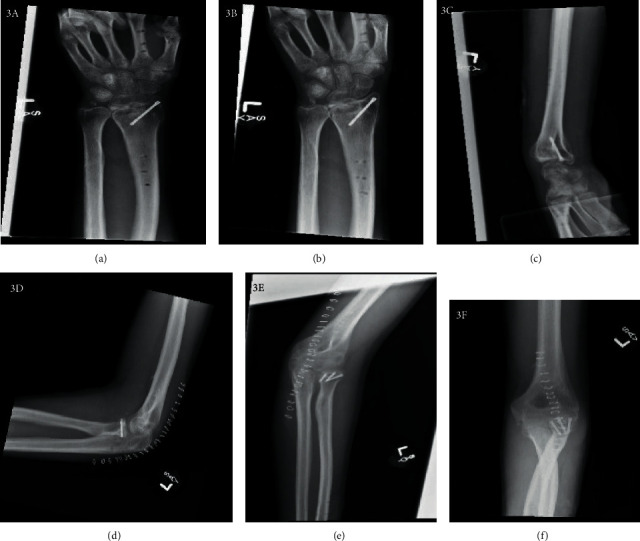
Radiographs at final follow-up showing healed fractures. Left wrist PA (a), external rotation (a) and lateral rotation (c). Left elbow lateral (d) and AP 2 views (e, f).
